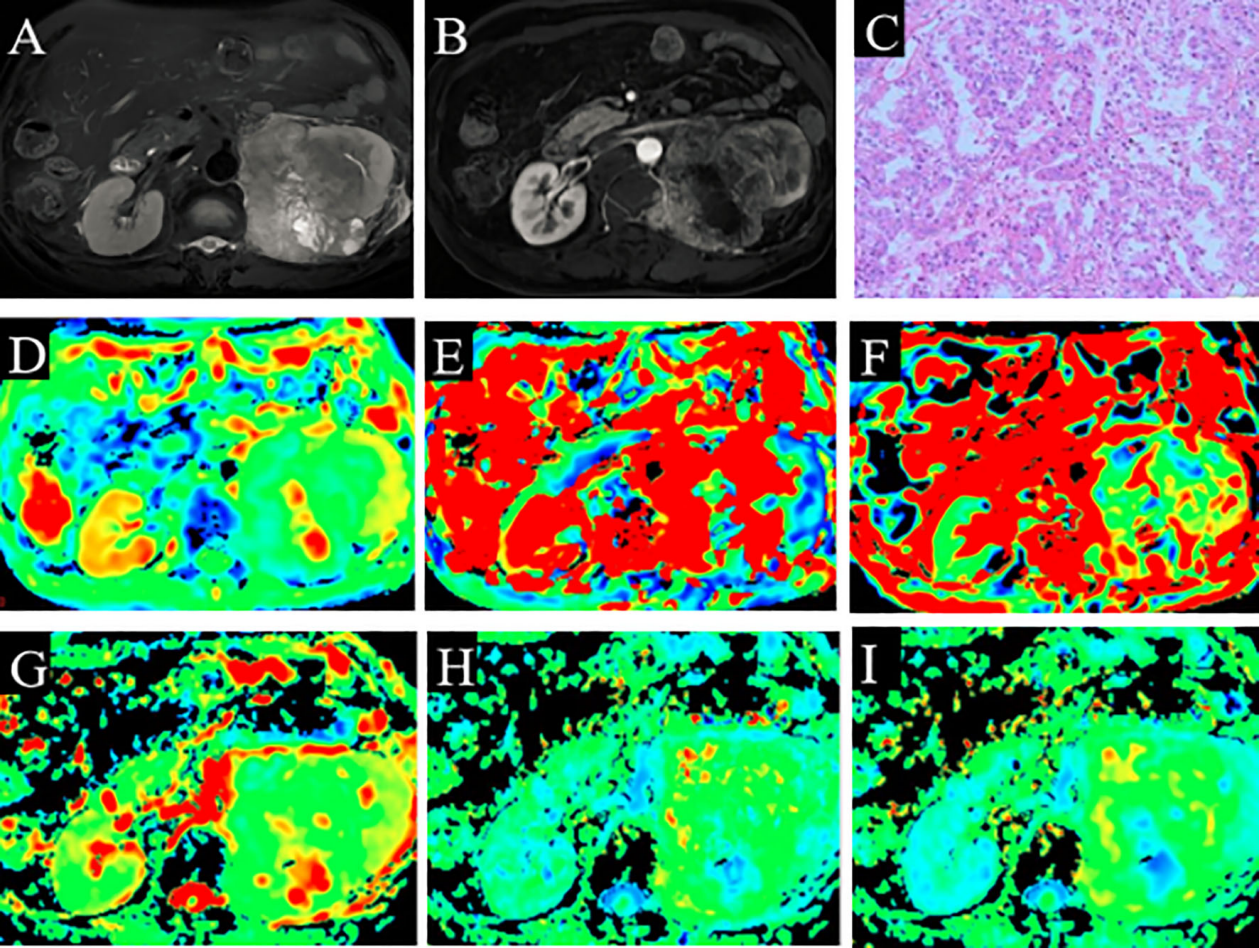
A 66-year-old man with ccRCC (grade 3 and stage 3). (A) High signal intensity on fat-saturated T2WI. (B) Solid part shows enhancement. (C) Pathological analysis confirmed ccRCC (grade 3). (D) D map. (E) D* map. (F) f map. (G) MD map. (H) KA map. (I) MK map. ccRCC, clear cell renal cell carcinoma; T2WI, T2-weighted imaging; MD, mean diffusivity; KA, kurtosis anisotropy; MK, mean kurtosis.
